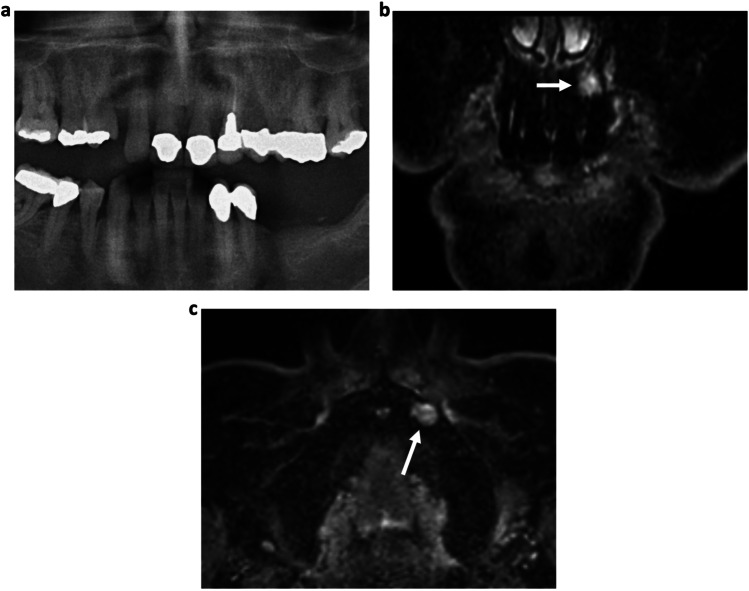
a OPT of a 67-year-old patient with root canal treatment of the tooth 21. Note that the roots of the teeth 12 to 21 are not assessable due to superimposition of the nasal cavity and sinuses. On the coronal (b) and axial (c) reconstructions of the 3D STIR sequence, a distinct periapical cyst/abscess (white arrows) can be detected next to the root of the tooth 21, which would have been missed on conventional radiography
